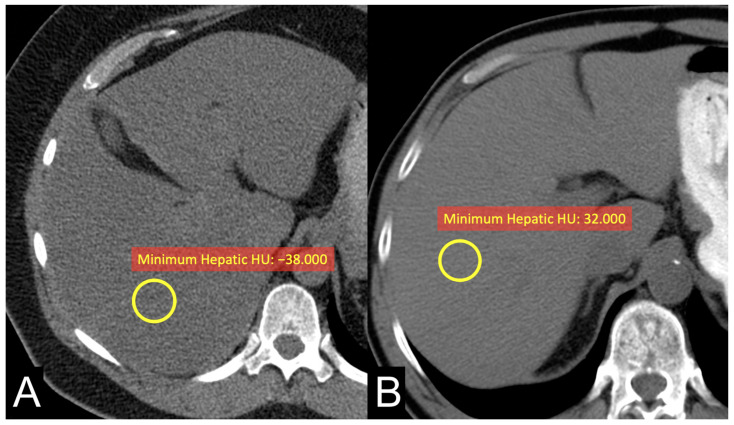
Unenhanced axial CT images of patients with low-grade ccRCC with ADFP expression (A) and low-grade ccRCC without ADFP expression (B) show yellow ROIs with different minimum hepatic HU values (HU −38 and 32, respectively).
